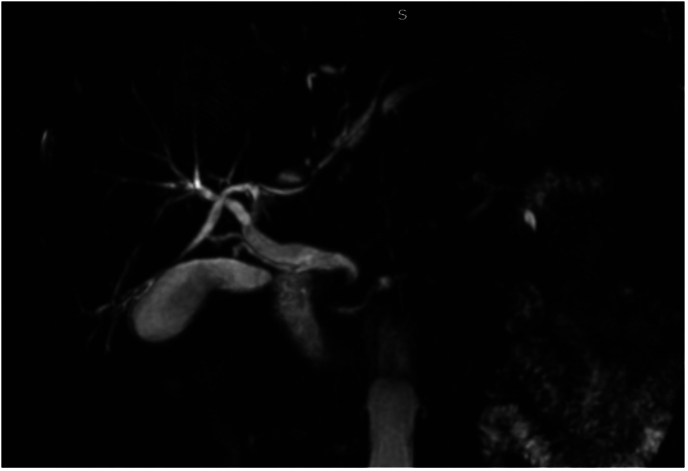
MRI imaging showing the hook-shaped distal CBD.
